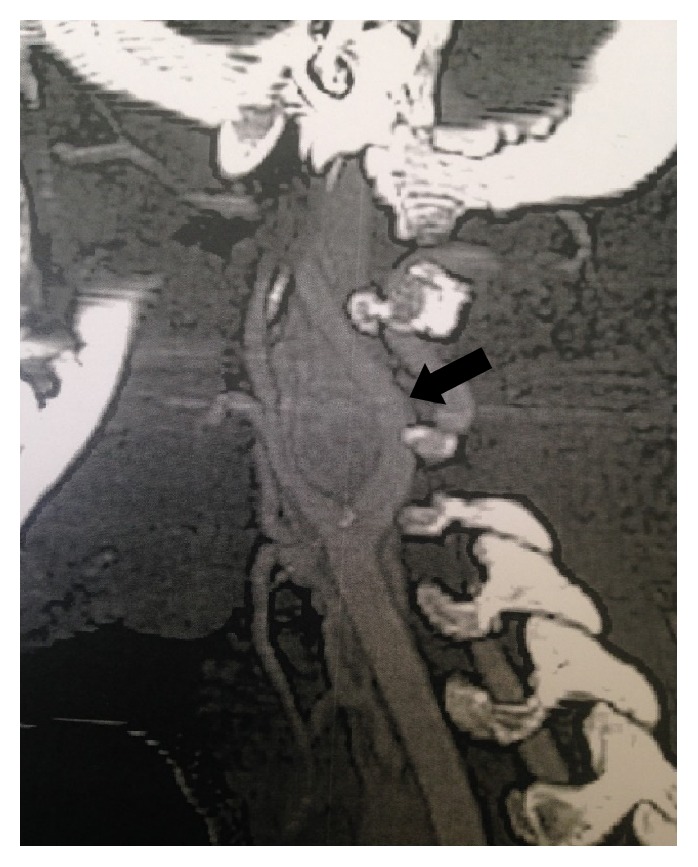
CTA-carotid body paraganglioma (CBP), lyre sign (arrow).
